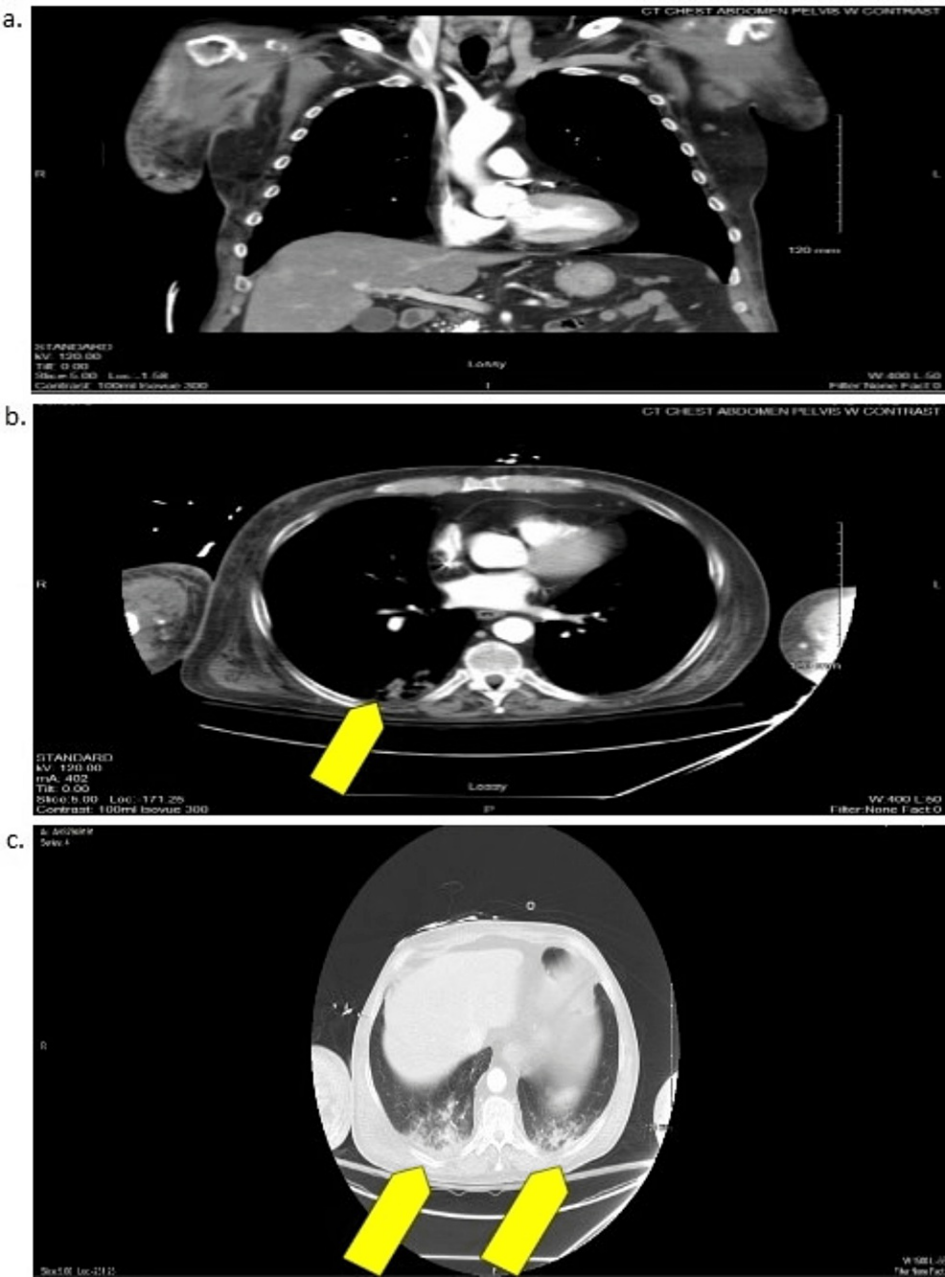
CT of the Chest With Contrast a: mid-coronal plane; b: mid, upper-transverse plane (yellow arrow indicates the area of consolidation); c: lower transverse plane, chest CT lung view (yellow arrows indicate areas of consolidation) CT: computed tomography
